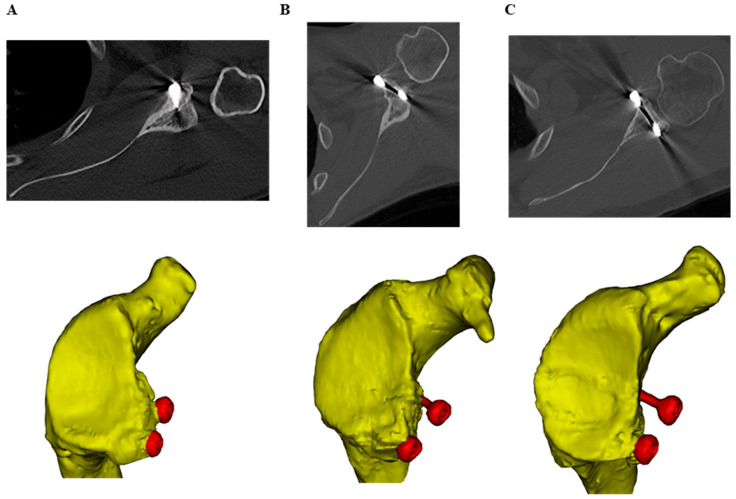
Resorption grades on the final follow-up axial CT scans, as defined by Zhu et al. (2015) [11], with the corresponding three-dimensional scapula geometries (yellow) and screws (red) generated by segmentation of CT scans of three different participants. (A) Grade I (minor resorption), (B) Grade II (major resorption) and (C) Grade III resorption (total resorption).
